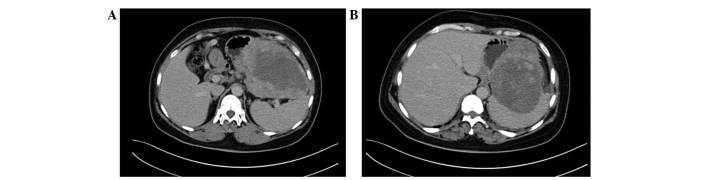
Abdominal computed tomography scan showed the large mass between the greater curvature of stomach and spleen, with (A) a number of enlarged lymph nodes adjacent and (B) invasion of the stomach and spleen.
